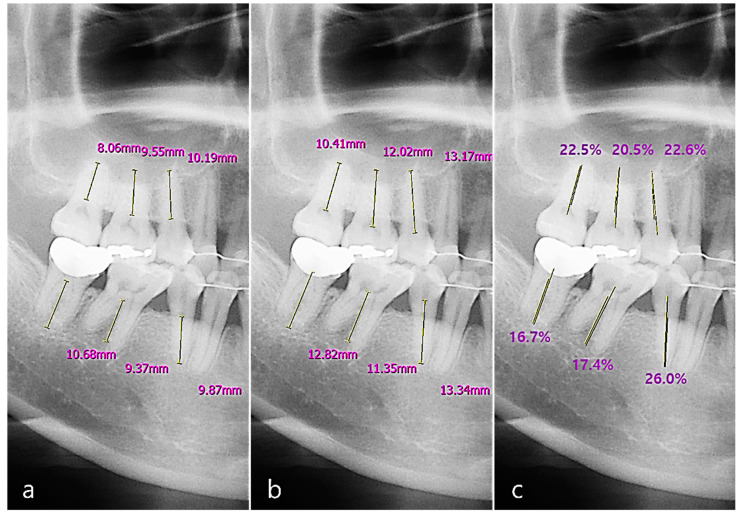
The measurement of RBL (radiographic bone loss) on a panoramic image: (a) the distance from the root tip of the tooth to the periodontal bone level (PBL); (b) the distance from the root tip of the tooth to the cementoenamel junction level (CEJL); (c) the percentage rate of the intersection length of the PBL and the other CEJL.
